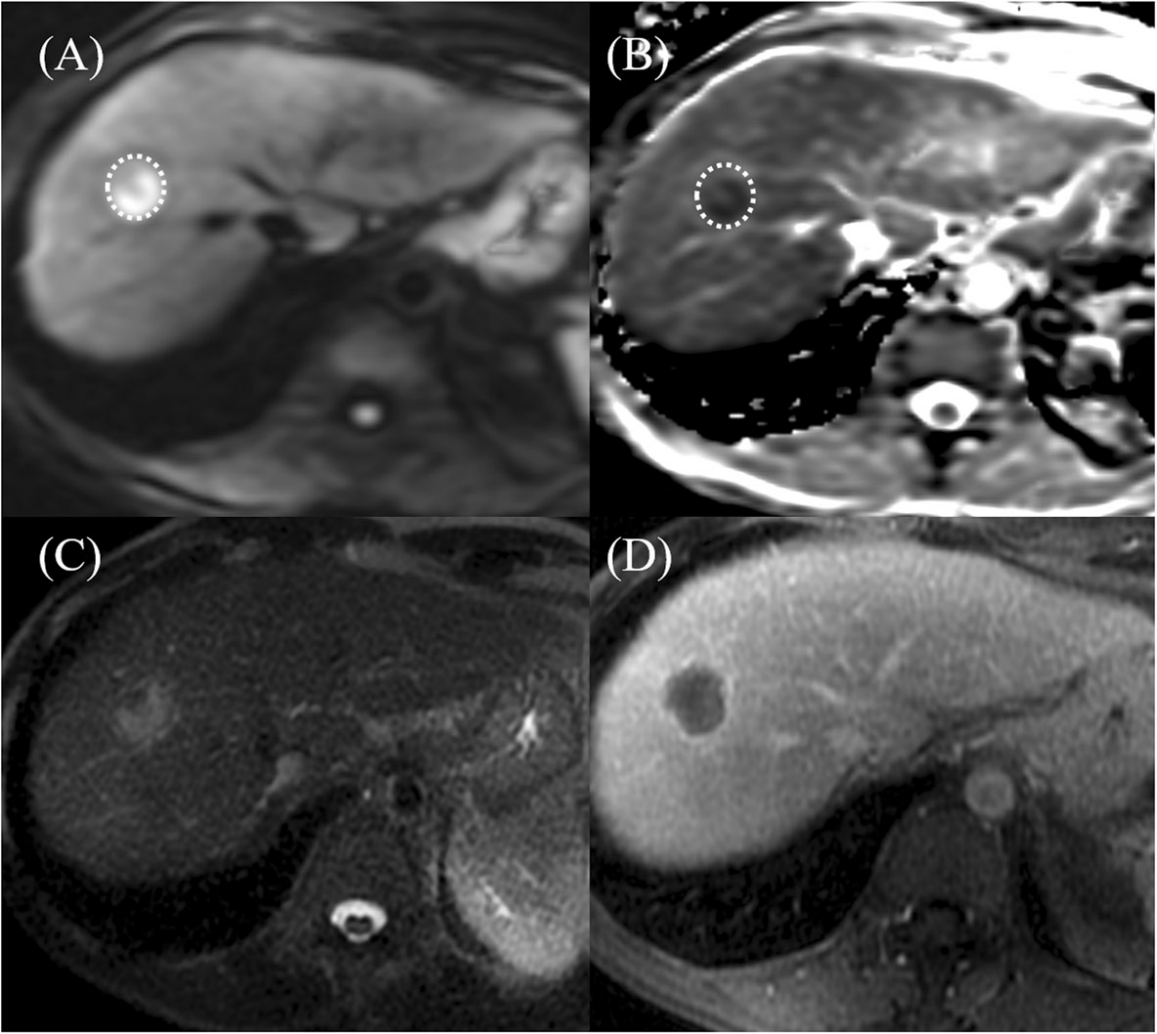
MR imaging in a 60-year-old man who underwent DEB-TACE procedure. Pathologically confirmed grade 4 HCC with 40% tumor necrosis. Dotted circles show the placement of regions of interest. a DWI and b ADC map show restricted diffusion area in the segment VIII tumor. c T2-weighted imaging. d Postcontrast T1-weighted image shows mildly enhancing viable tumor. ADC_min_ of tumor was 0.84 × 10^− 3^ mm^2^/s
